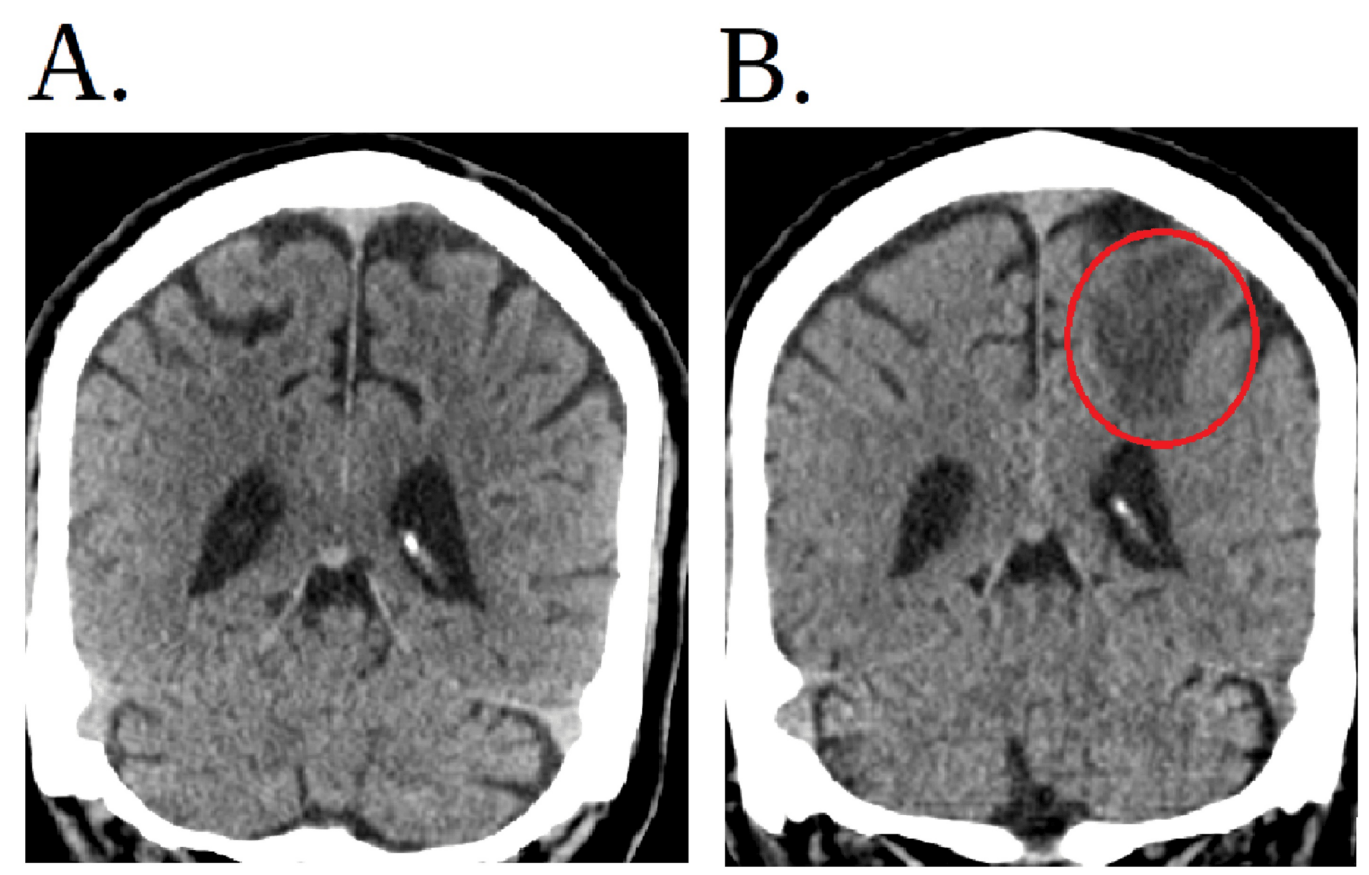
CT of the brain and head (A) Initial CT scan that was done in the emergency department at first presentation when the patient had his trauma from falling from his roof which shows no metastasis. (B) Repeat CT of the brain/head without contrast approximately one year later shows probable left parietal lobe intra-axial metastasis with associated vasogenic edema noted, new since the initial CT scan of the brain. CT: computed tomography
